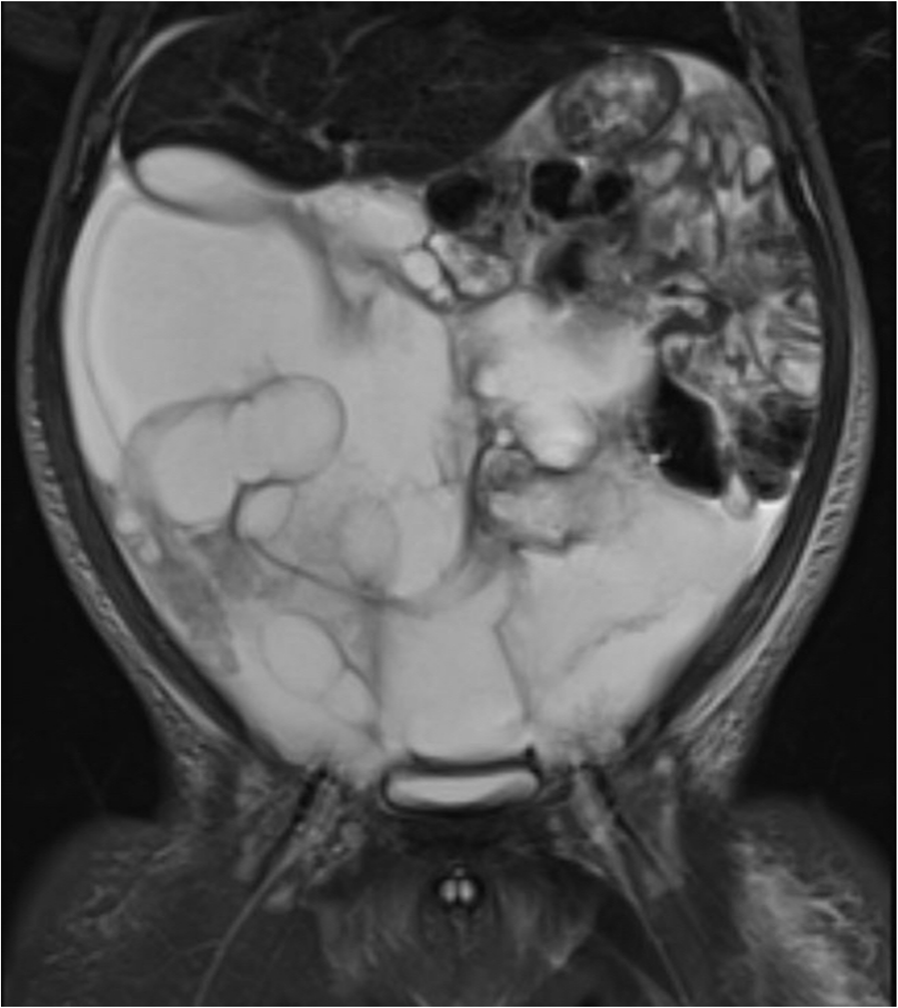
Coronal T2 weighted MR image of a large mesenteric lymphatic malformation in a 2 year old male. This lesion would be suitable for serial sclerotherapy via 2–3 pigtail drains
